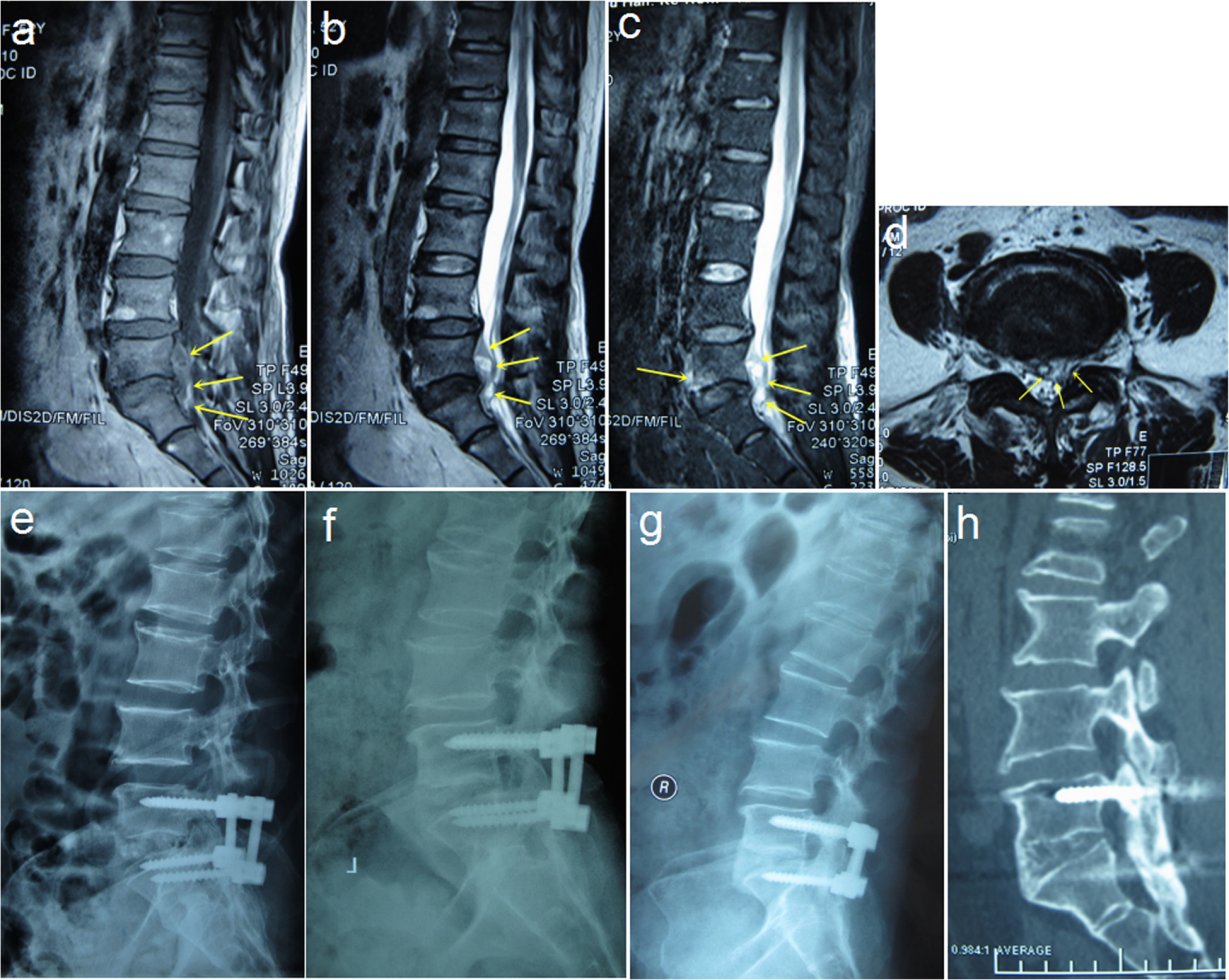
A 52-year-old female misdiagnosed as “disc herniation” at the first visit. After 4-month conservative treatment, the clinical symptoms aggravated. a, b, c T1,T2-weighted and STIR MRI showed inflammation in L5–S1 vertebral bodies and intervertebral disc. Spinal epidural abscess and inflammatory granuloma extend to anterior epidural space, resulting in spinal stenosis at L5-S1 level; (d) Transverse T2-weighted MRI showed left nerve root and dural sac compression; (e) postoperative X-ray showed intervertebral bone grafting and instrumentation; (f, g) 6-month and 36-month postoperative X-ray showed solid bony fusion. (h) the last follow-up CT showed solid intervertebral and facet joint bony fusion
